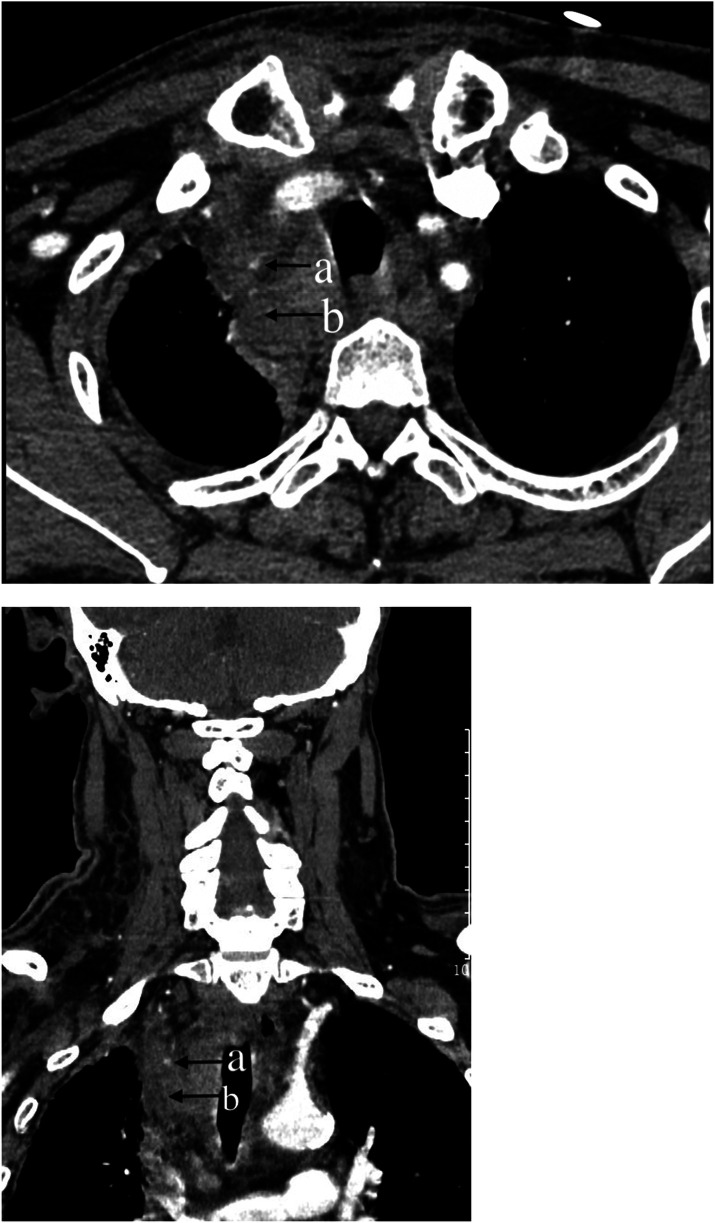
Chest computer tomography (CT) scan of the neck and chest: (a) Dot-like enhancement in the arterial phase, indicating active bleeding. (b) Mediastinal hematoma.
